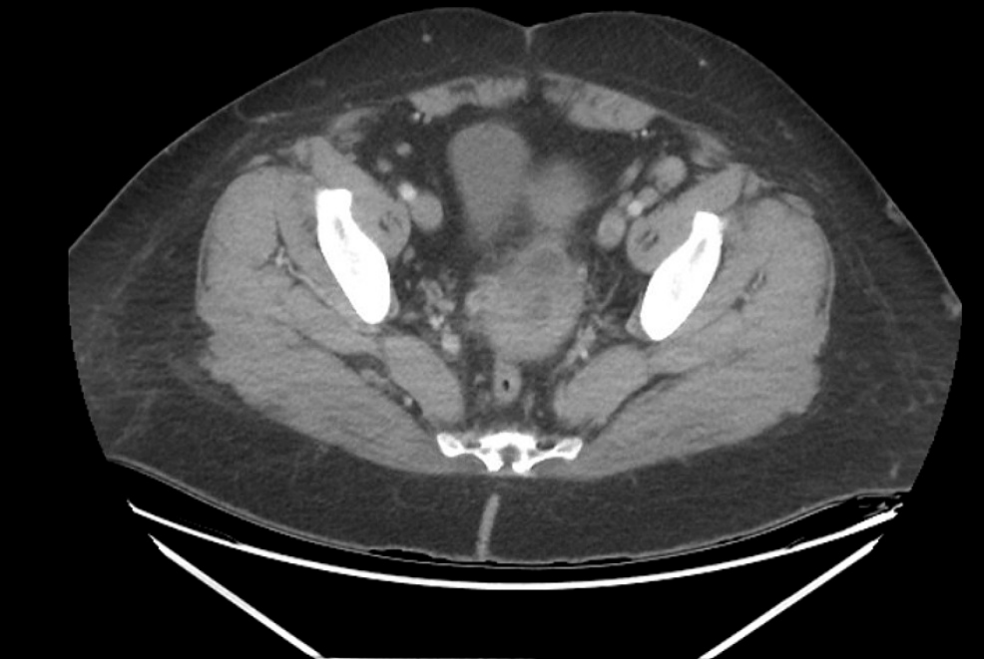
Axial CT image of the abdomen/pelvis with IV contrast demonstrating no hematoma. CT, computed tomography
